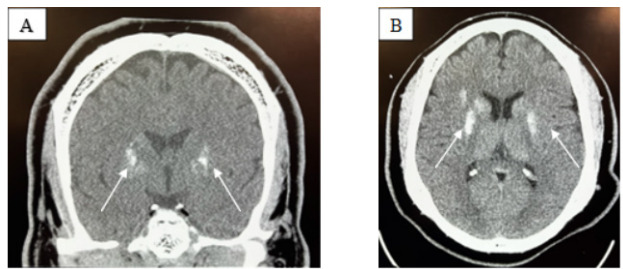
Non-contrast computed tomography of the head: (A) coronal view, and (B) axial view, demonstrating bilateral basal ganglia calcifications (white arrows) in a patient with Fahr disease.
